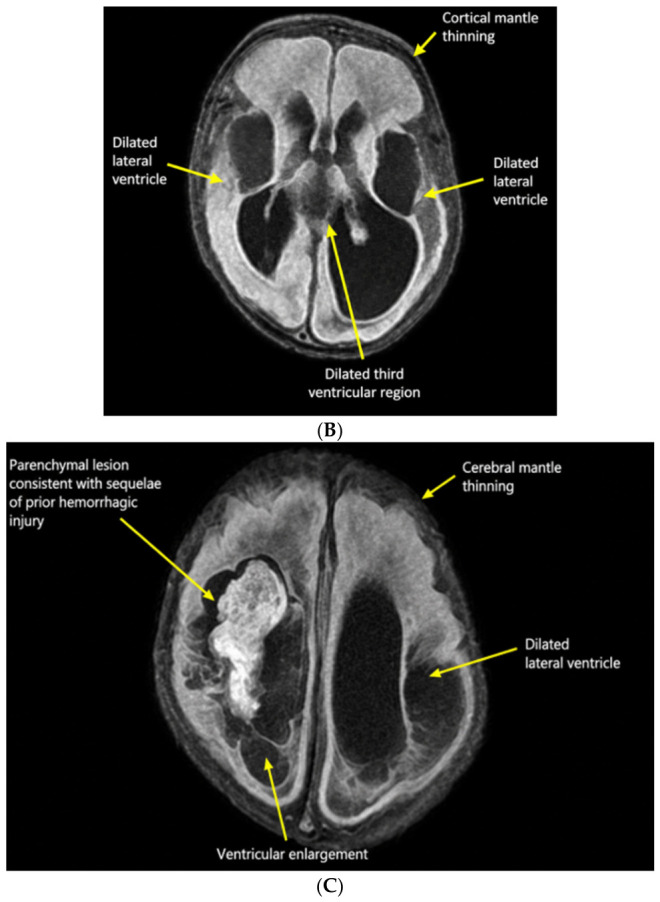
Representative brain MRI images obtained on 21 May 2025. (A) Severe ventriculomegaly with marked dilatation of the lateral ventricles and pronounced cerebral mantle thinning. (B) Additional axial section confirming extensive supratentorial ventricular enlargement with distortion of adjacent cerebral structures. (C) Associated parenchymal abnormalities, including a right frontoparietal hemorrhagic lesion and surrounding changes consistent with sequelae of prior hemorrhagic brain injury in the setting of neonatal intraventricular hemorrhage. The MRI findings demonstrated severe ventricular enlargement, intraventricular hemorrhagic sequelae, and associated parenchymal injury. However, because dedicated cerebrospinal fluid flow sequences were not performed, MRI could not definitively establish the exact site or mechanism of cerebrospinal fluid pathway obstruction. (A) Axial brain MRI demonstrating marked bilateral ventriculomegaly with severe dilatation of the lateral ventricles, enlargement of the frontal horns, and pronounced thinning of the surrounding cerebral mantle. (B) Additional axial brain MRI section demonstrating marked enlargement of the supratentorial ventricular system, including severe bilateral lateral ventricular dilatation, distortion of adjacent cerebral structures, and associated cerebral mantle thinning consistent with advanced post-hemorrhagic ventricular dilatation. (C) Axial MRI demonstrating parenchymal abnormalities adjacent to the ventricular system, including a right frontoparietal hemorrhagic lesion and surrounding changes consistent with sequelae of prior hemorrhagic brain injury in the setting of neonatal intraventricular hemorrhage.
